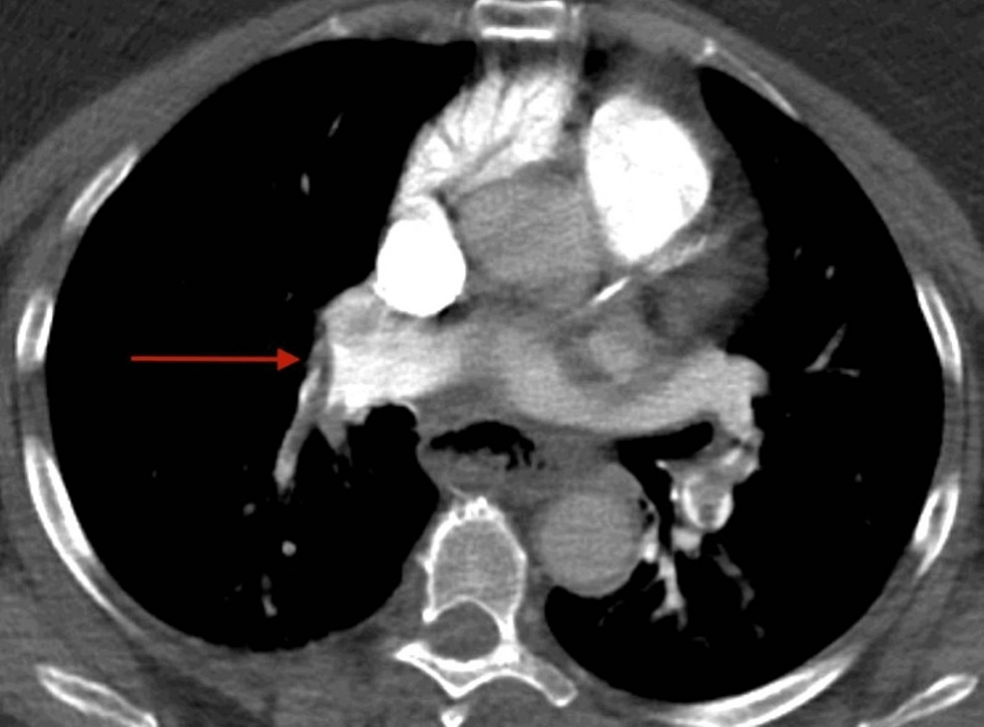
CT pulmonary angiogram showing a red arrow pointing at a pulmonary embolus in the right pulmonary artery. CT, computed tomography
